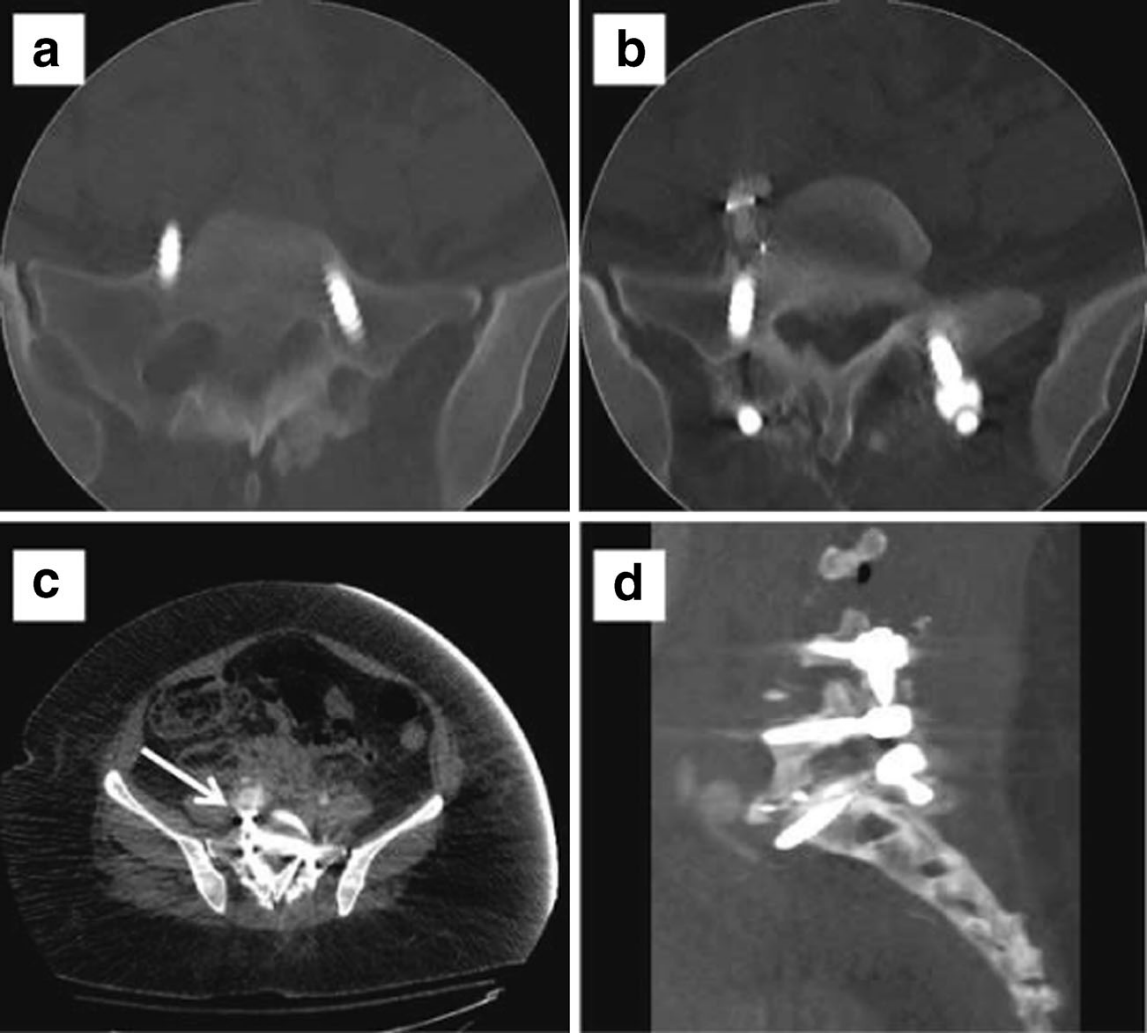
Status post L4–5 and L5–S1 fusion. Spacer cage is displaced anterolaterally to the right with a right S1 screw breaching the anterior cortex on axial CT images. a and b Axial CT slice through the lower abdomen shows retroperitoneal haemorrhage and right internal iliac artery pseudoaneurysm (*arrow*), abutting the displaced cage on sagittal reformats (d)
